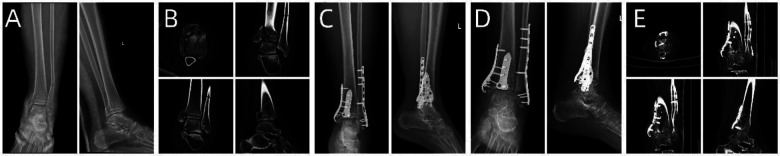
Patient's preoperative and postoperative radiological data. (A,B) Preoperative x-ray and CT images. (C) x-ray image on the first day after surgery. (D,E) x-ray and CT images 6 months after surgery.
